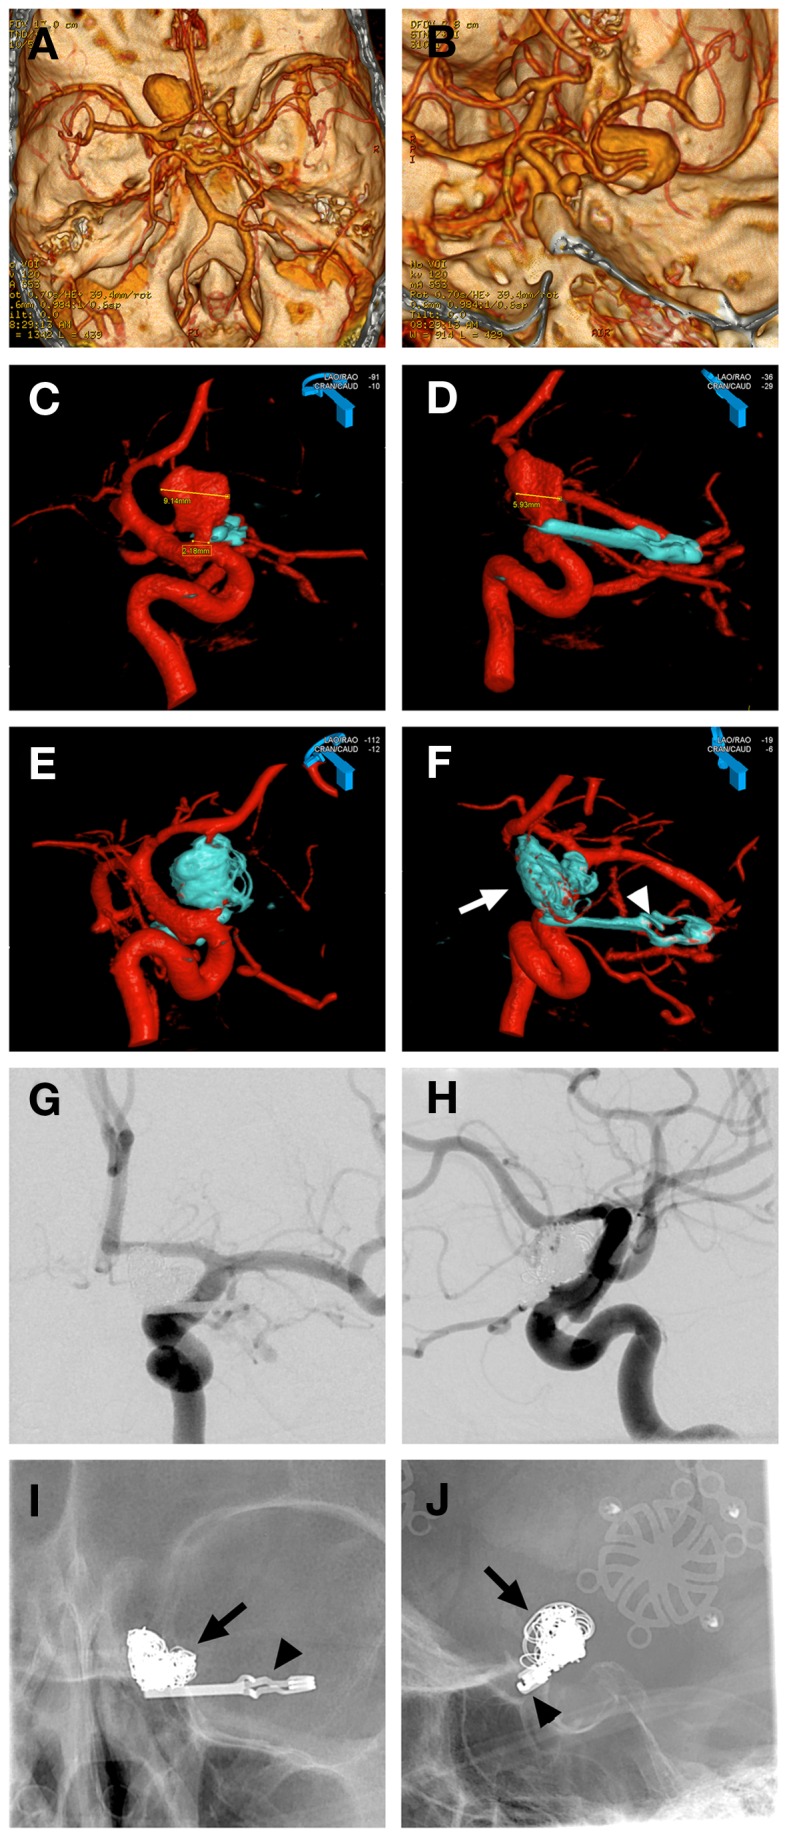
Case 3. (A,B) CT angiographic 3D reconstruction views showing the left giant carotid ophthalmic artery aneurysm; (C,D) 3D angiographic reconstructions from left ICA showing the left carotid ophthalmic artery aneurysm partially obliterated post clipping with a reconstructed narrow neck by the microsurgical clip (blue); (E,F) 3D angiographic reconstructions from left ICA post clipping and coiling with the giant aneurysm completely obliterated by the microsurgical clip (arrowhead) and the coil mass (arrow); (G,H) AP and lateral magnified subtracted left ICA angiographic views showing complete obliteration of aneurysm with clip and coils, ophthalmic artery is patent and well visualized (H); (I,J) AP and lateral magnified skull x-rays showing the microsurgical clip (arrowhead) and coil mass (arrow).
